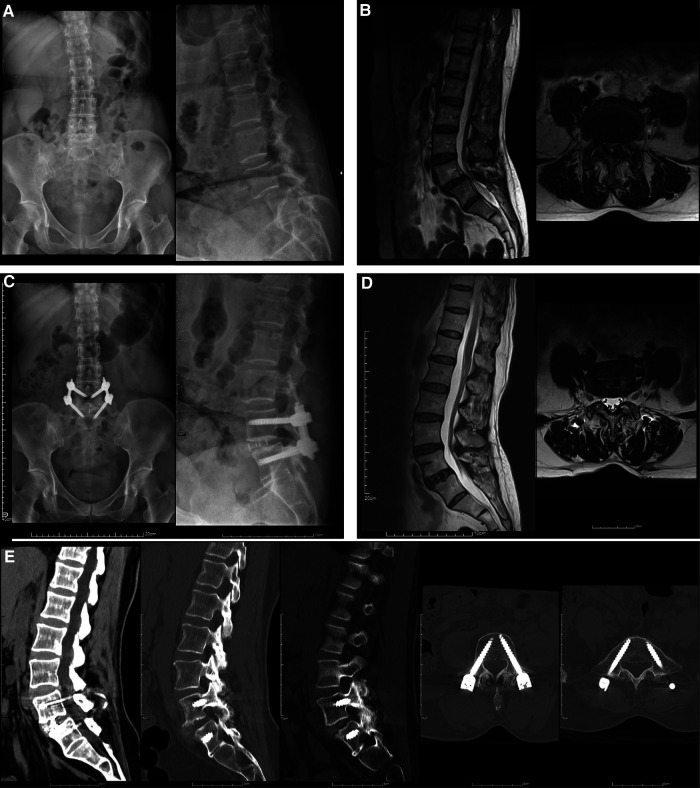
Preoperative and postoperative images of a 55-year-old woman with L4-5 spondylolisthesis grade II who underwent two-stage OLIF. (A) Preoperative radiographs. (B) Preoperative and MRI. (C) One-month postoperative radiographs. (D) 15-Month postoperative MRI and (E) 12-Month follow-up CT scan showed ankylosis of bilateral facet joint.
